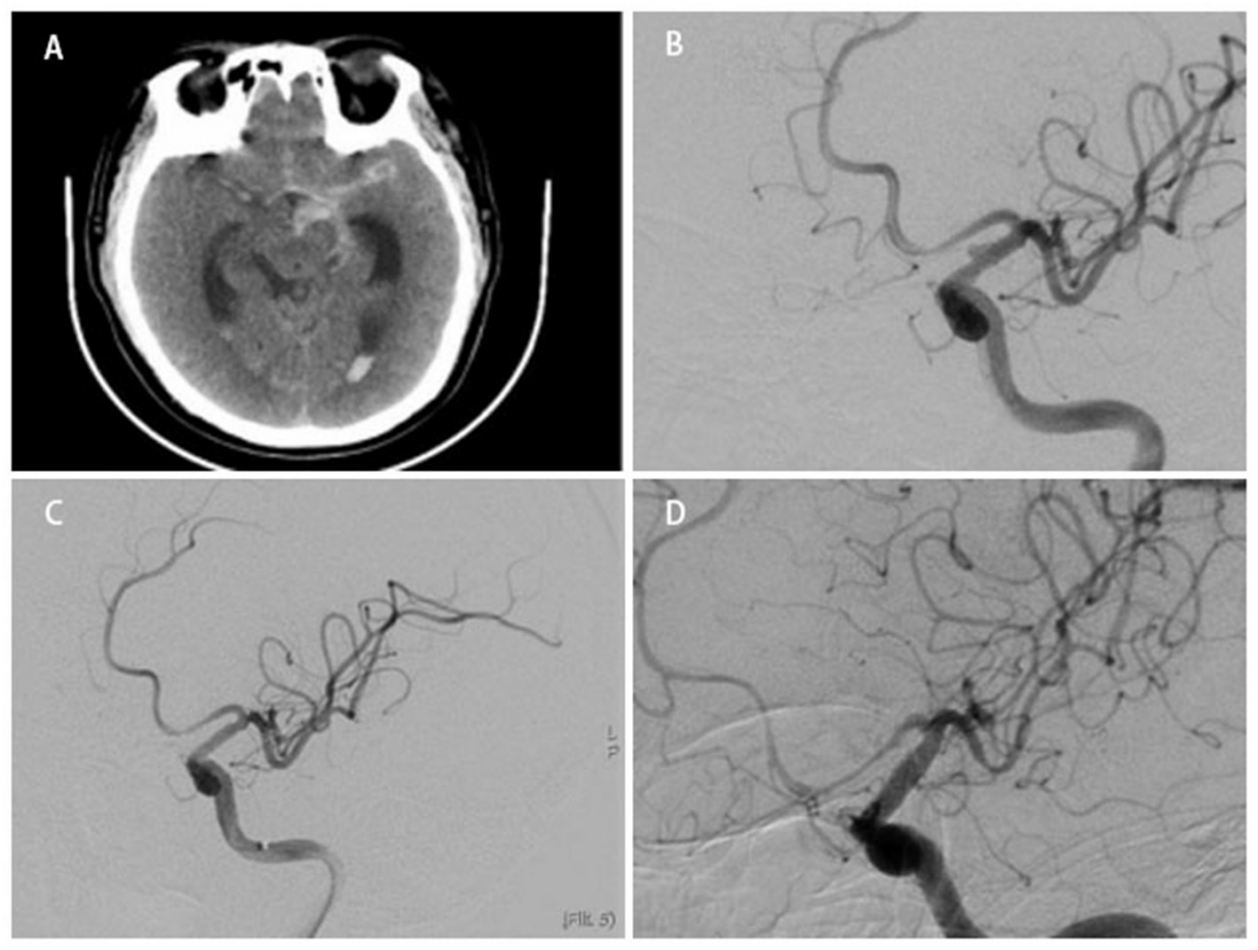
A middle-aged person was admitted to our hospital for sudden headache 8 h earlier (H-H grade 2). An emergency cranial CT revealed diffuse subarachnoid hemorrhage (A). Cerebral angiography showed a BBA located at the side wall of the C7 segment of left ICA (B). After a WCS (3.5 ^*^ 13 mm) deployed successfully, the instant angiographic after once balloon dilation revealed no contrast filling into the aneurysm (C). Angiographic follow-up at 4 months revealed total occlusion of the aneurysm (D).
